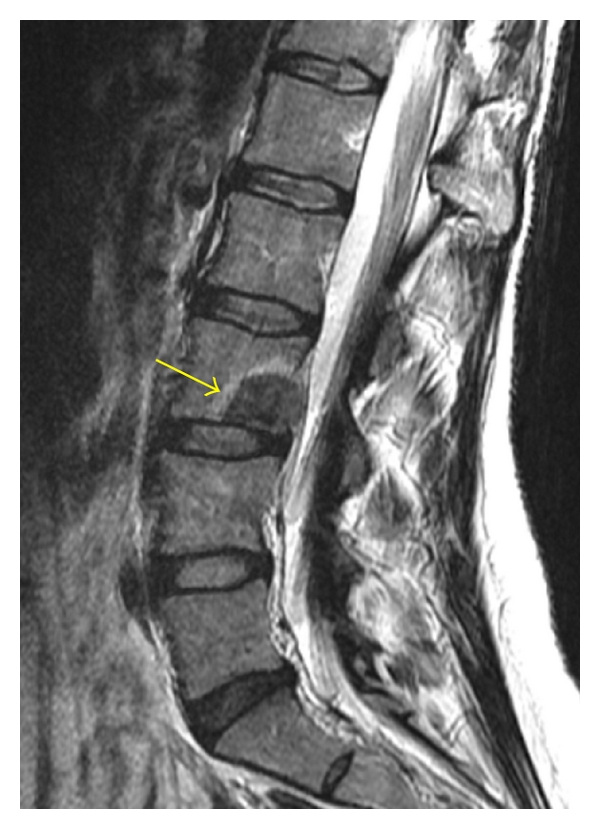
Sagittal T2-weighted MR image depicts the “halo sign” with a hypointense metastatic lesion and a surrounding hyperintense rim in the L3 vertebral body (yellow arrow).
